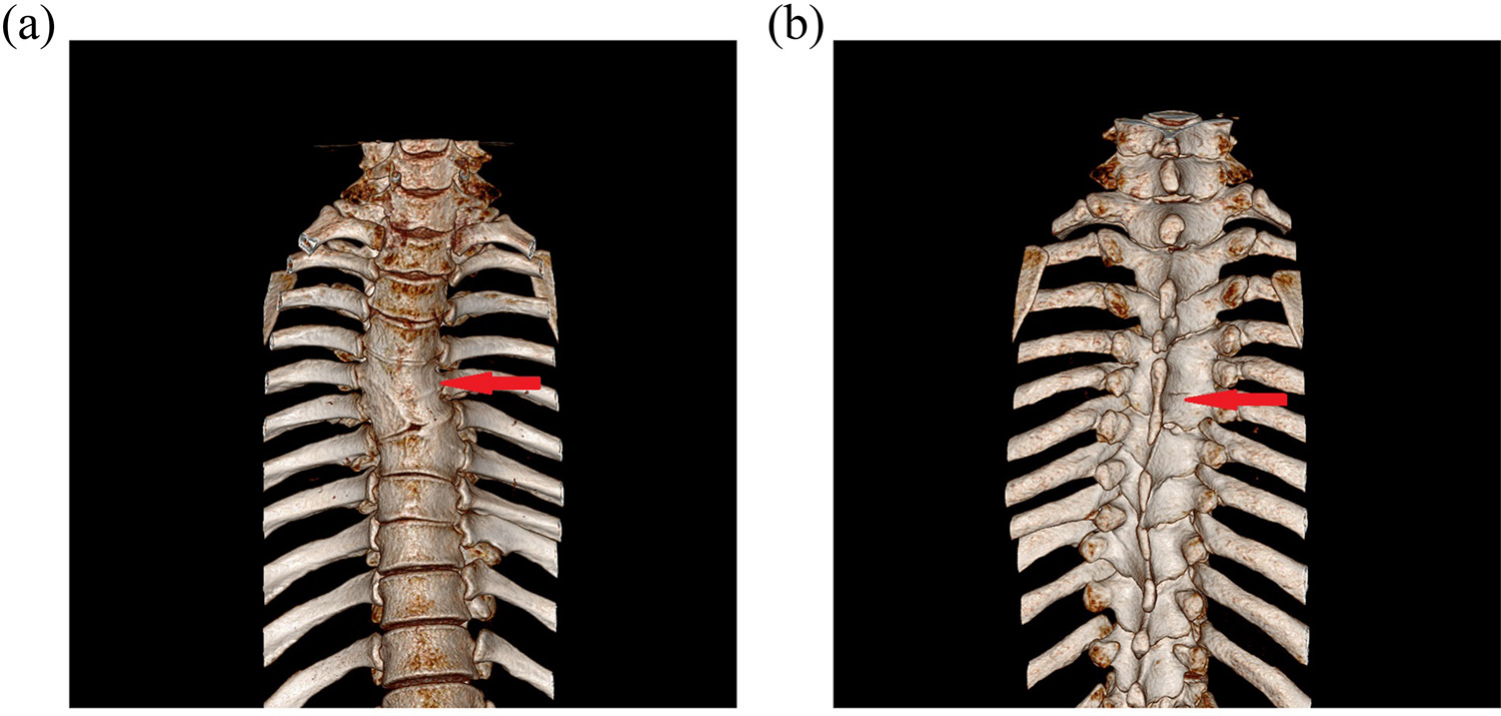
(a) and (b) CT three-dimensional reconstruction of the third to the fourth thoracic vertebrae with spinous process fusion.
